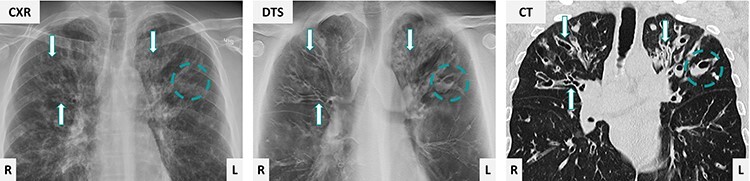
An adult patient with CF examined by CXR, DTS and CT. Pathological changes such as wall-thickened bronchiectasis (arrows) and mucus plugging (circles) are difficult to delineate on CXR because of overlapping anatomy, though clearly visible on DTS and CT.
